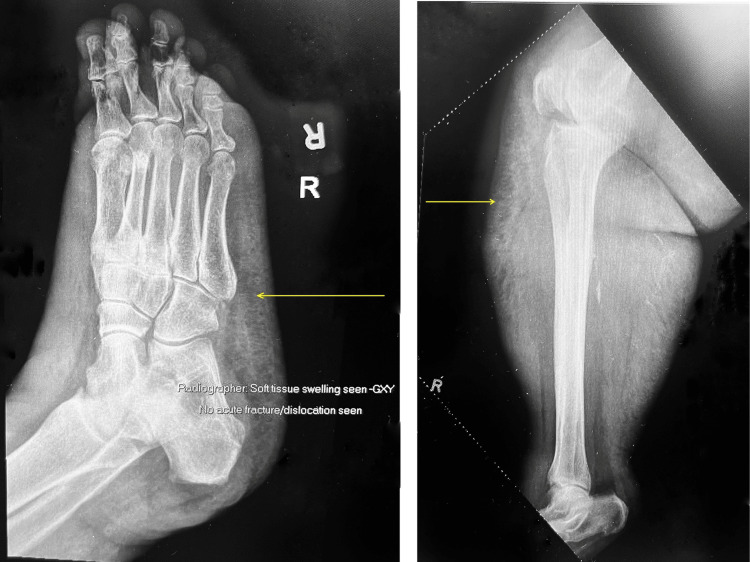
Right lower limb X-rays Subcutaneous gas and soft tissue swelling are marked by the yellow arrows. These findings support the diagnosis of necrotizing fasciitis.
